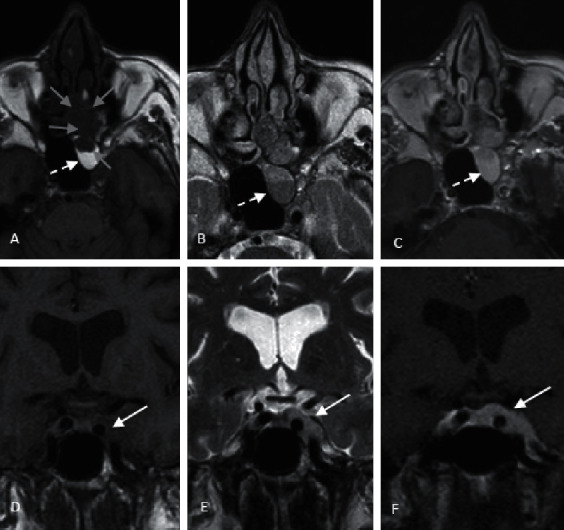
Initial MRI images: (a, c) axial and coronal T1WI, (b, e) axial and coronal T2WI, and (c, f) axial and coronal T1WI after gadolinium intravenous administration. The left nasal, ethmoidal, and sphenoidal lesion (grey arrows) can be seen with cavernous extension (solid white arrows), with low signal on T1WI and T2WI and homogeneous enhancement after contrast administration, with sinus obstruction and retention of secretions in ethmoid and sphenoid sinuses (dashed white arrows). MRI, magnetic resonance imaging; WI, weighted image.
